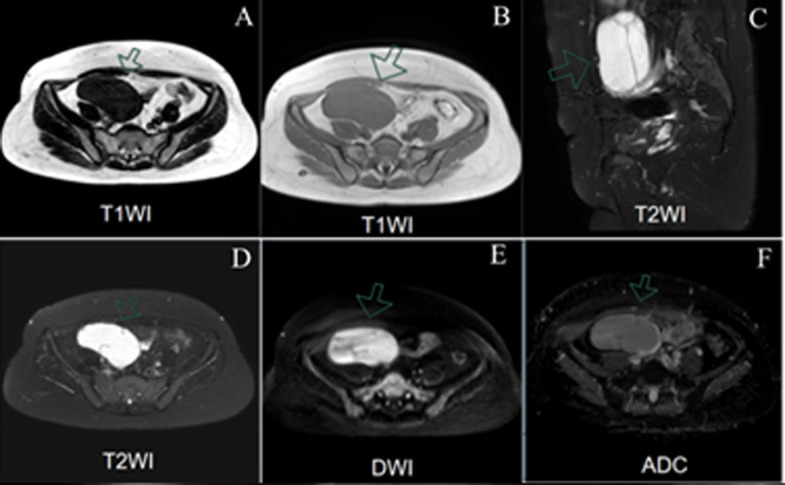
pelvic MRI findings of a right adnexal mass; A, B) T1-weighted imaging (T1WI) showing a well-defined mass with smooth margins and homogeneous intermediate signal intensity in the right adnexa; C, D) T2-weighted imaging (T2WI) reveals a hyperintense mass containing hypointense septations; E, F) Diffusion-weighted imaging (DWI) showing high signal intensity with slightly reduced ADC values, indicating mildly restricted diffusion
